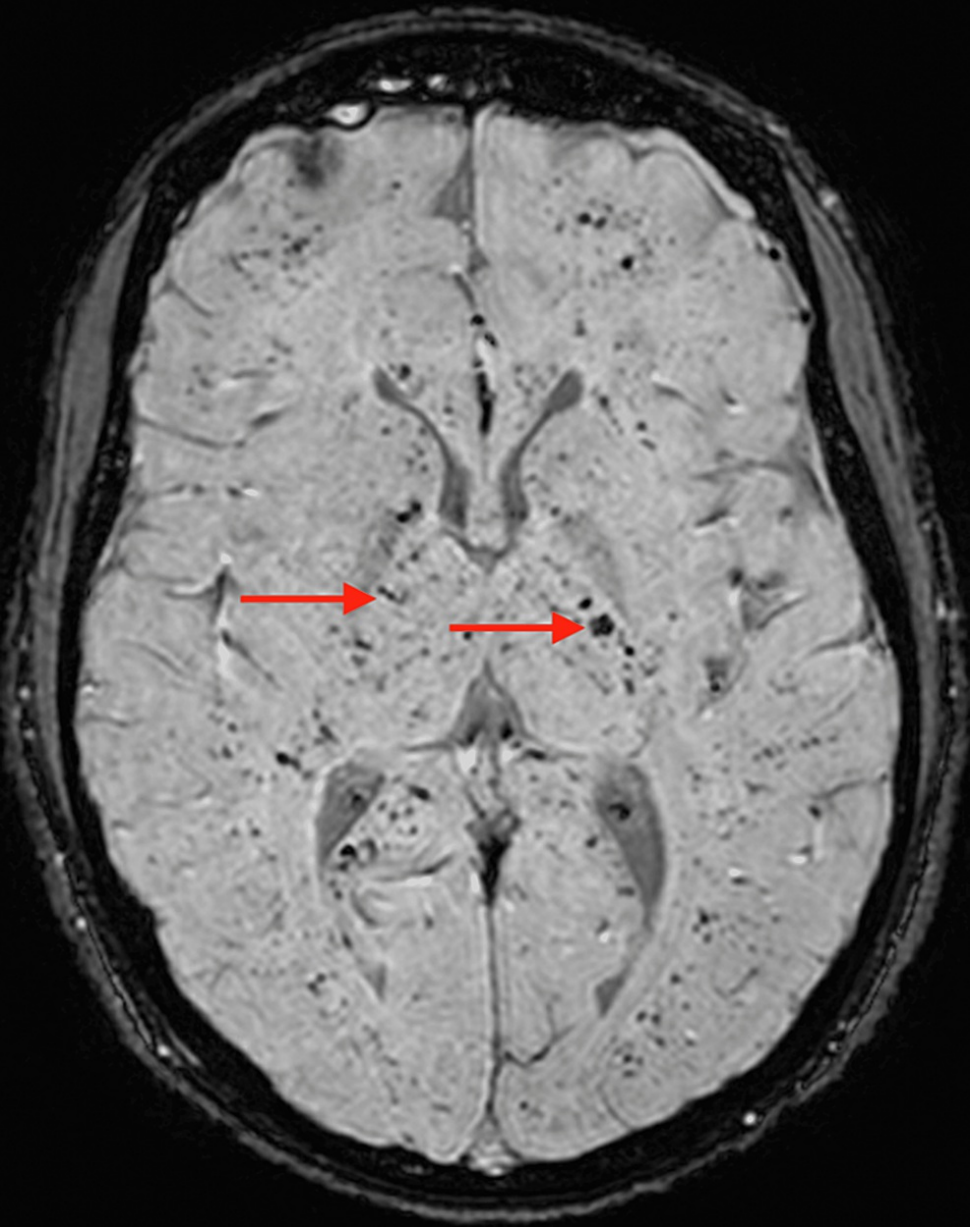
Axial MRI SWI sequence showing innumerable foci of signal attenuation in the descending cortical tracts consistent with “starfield” appearance of fat embolism
